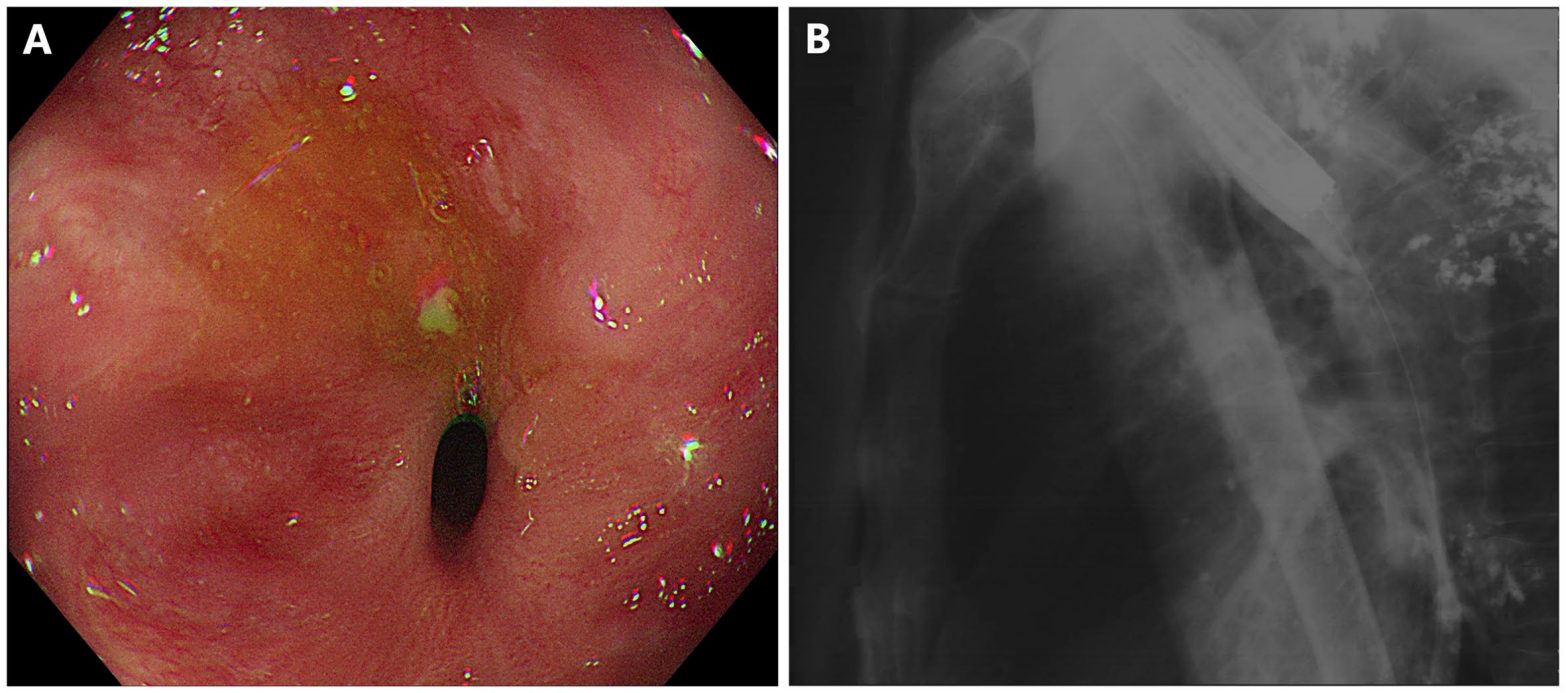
Short-segment anastomotic stricture and fistula in a patient with esophageal carcinoma after esophagectomy. (A) Esophagogastro-anastomosis stricture with an inner diameter of 3 mm. (B) X-ray radiography showed anastomotic leak connected to the right bronchus.
